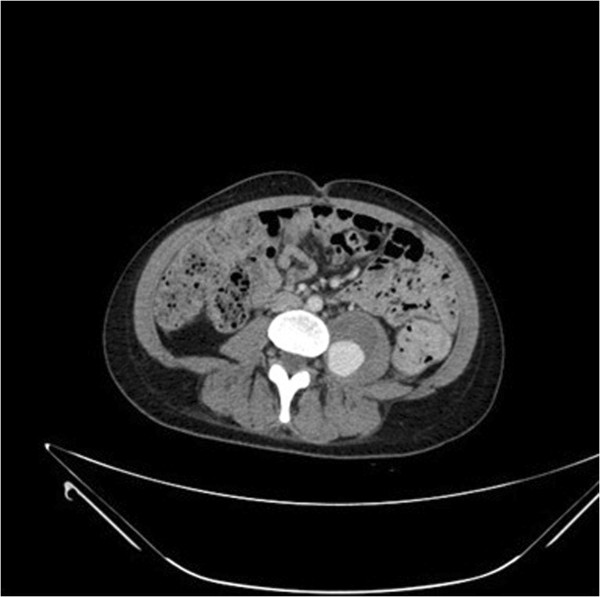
CT Angiogram with signs of embolisation of the pseudoaneurysm of the left lumbar artery L4, with filling of the aneurismal sac.
